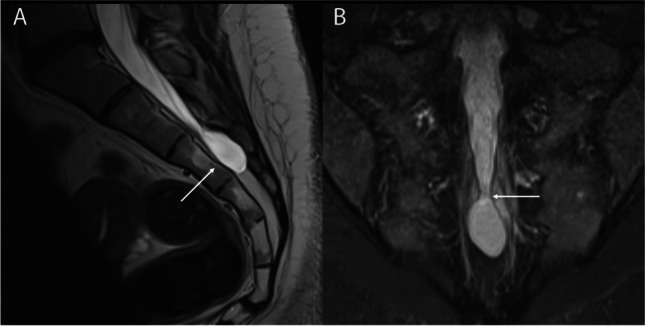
Sacral meningeal diverticulum in a 33-year-old patient. A T2-weighted sagittal image of the sacrum shows the meningeal diverticulum enlarging the sacral canal at the S3 level (arrow). B Coronal STIR demonstrates the wide-neck of the diverticulum (arrow) across which CSF is in free communication with the subarachnoid space
